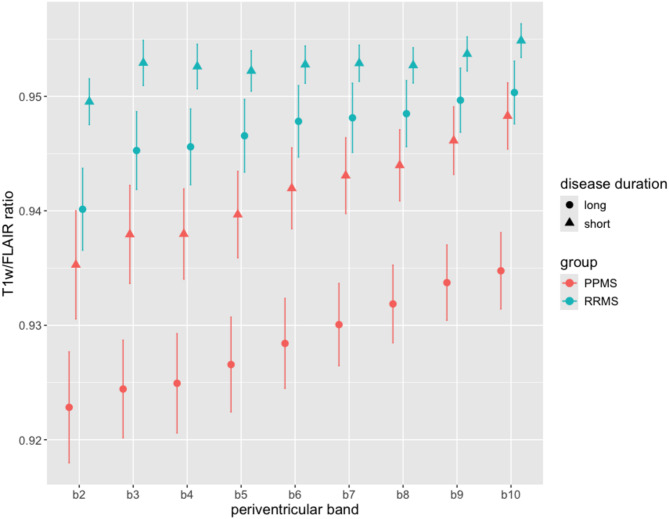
T1w/FLAIR ratios for long and short disease durations. Mean T1w/FLAIR ratios in each periventricular band (band b2 to band b10) for relapsing-remitting MS (RRMS) and primary progressive MS (PPMS) patients divided into long (>10 years) and short (<10 years) disease durations. Error bars represent standard errors
